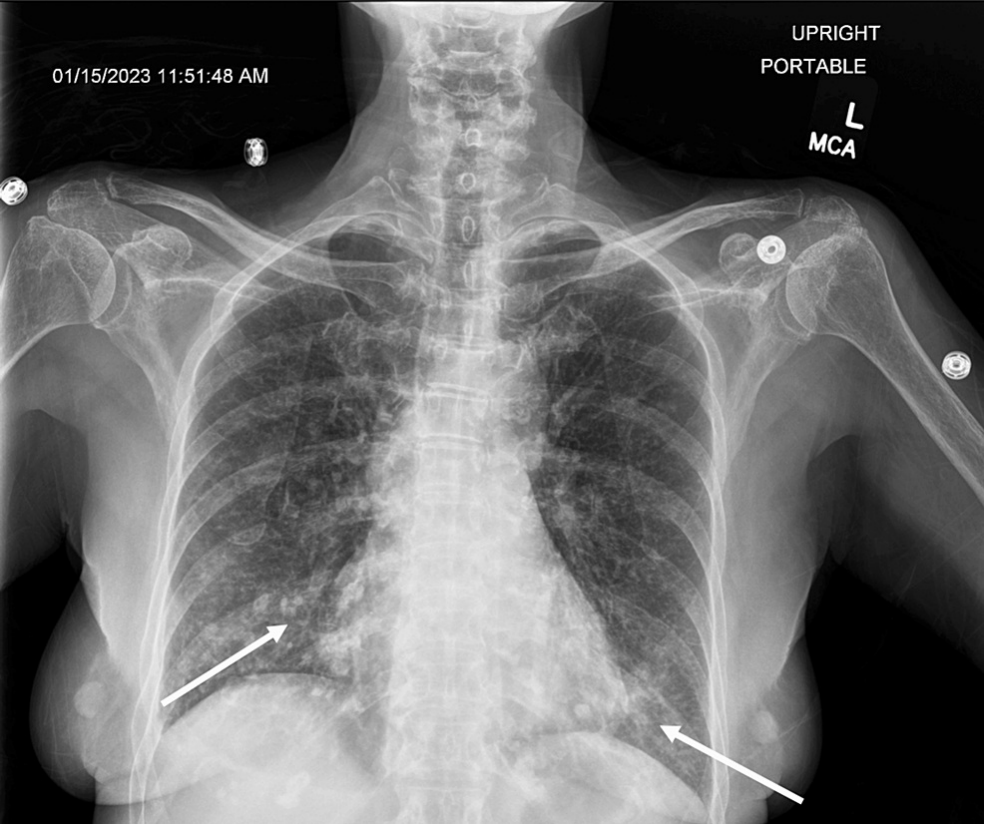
Chest X-ray revealing millet seed-sized tuberculosis foci in bilateral lungs
